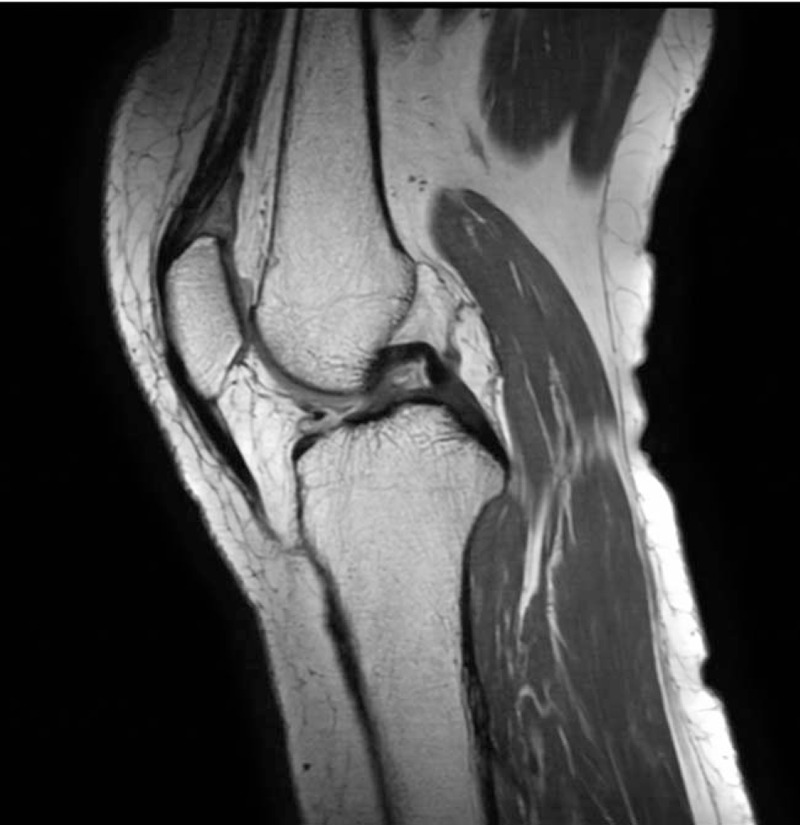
Left knee magnetic resonance image of the edematous change of the suprapatellar fat pad with increased signal intensity in T2-weighted image and posterior bulging contour of the fat pad (suggesting suprapatellar fat pad edema syndrome), and an irregular low signal line with inner high signal at the subchondral portion of the lateral femoral condyle (posterior aspects).
